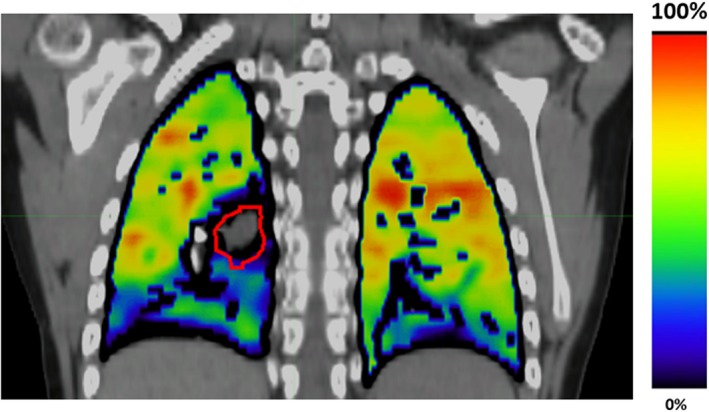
An example of a 4DCT‐ventilation image overlaid with a standard CT. The bright colors represent function lung while the darker tones display areas of lower functioning lung. The displayed patient presents with a mass (outlined in red) that is occluding an airway and is causing a defect in the right lower lobe.
